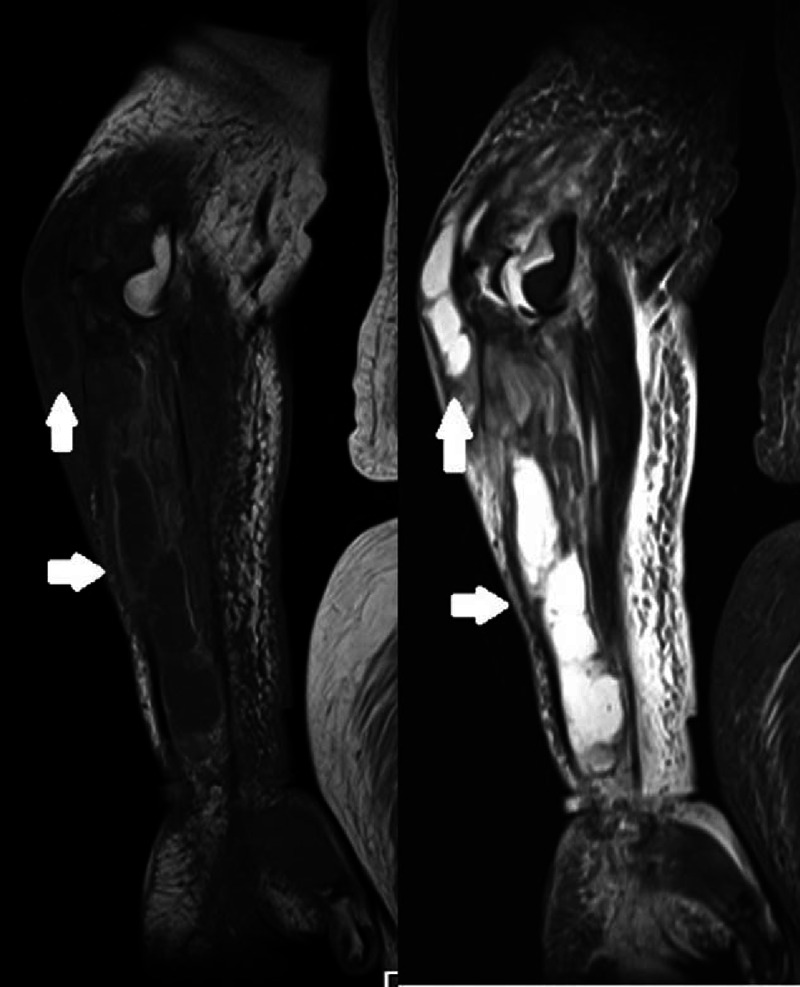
Magnetic resonance imaging shows fluid collection in the right elbow joint and forearm (arrows).
